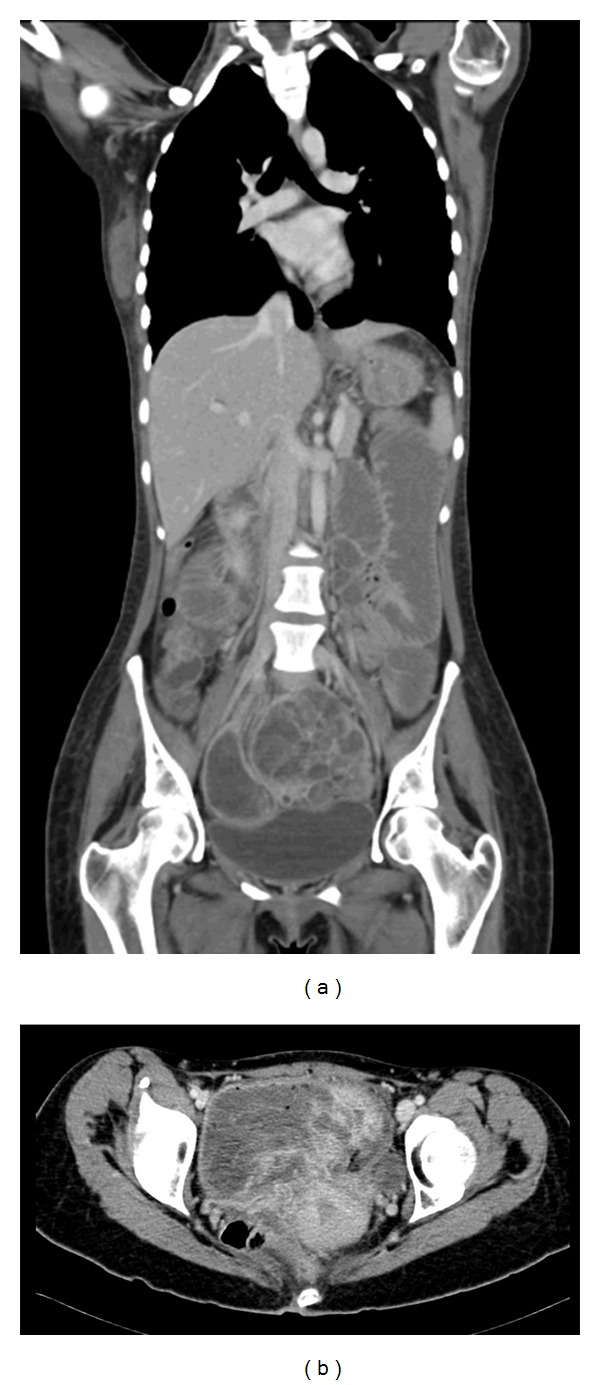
Computed tomography (CT) of the abdomen. (a) Coronal section and (b) axial section showing a huge 12.8 × 10.2 × 13.1 cm heterogeneous, aggressive-looking intraperitoneal pelvic mass lesion, most likely arising from intestinal ileum, with areas of lobulations, hemorrhagic cystic changes, gas locules, fluid-fluid levels, and proximal small bowel dilatation due to partial obstruction.
